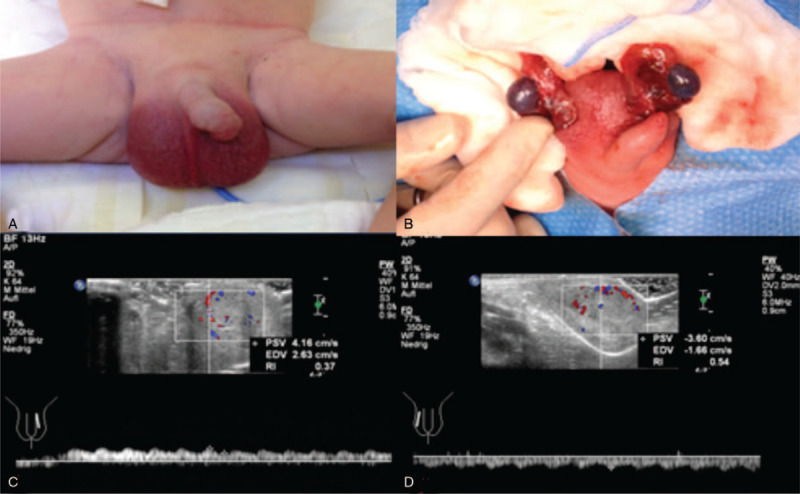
(A) Preoperative situation with scrotal discoloration and swelling, (B) intraoperative finding after detroquing and warm packing. The testicles remain dark with no signs of reperfusion. (C) (right), (D) (left): Postoperative doppler ultrasound of both testicles, showing good perfusion on both sides.
